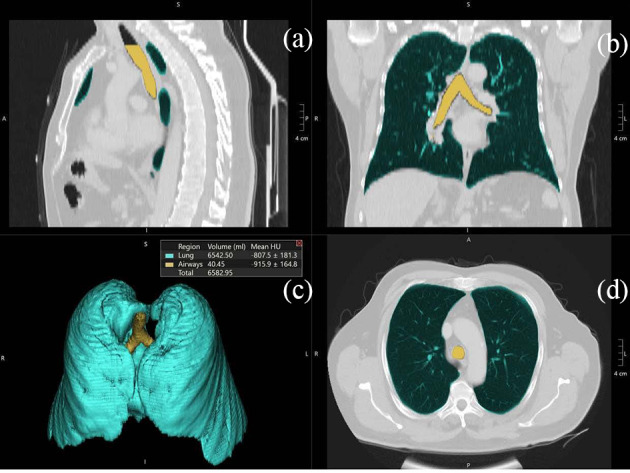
CT-based volumetric assessment of total lung parenchyma and airway volumes in sagittal (A), coronal (B), three-dimensional volumetric reconstruction images demonstrating calculated total lung parenchymal and airway volumes (C), and axial (D) images. Figure 2 long description.
